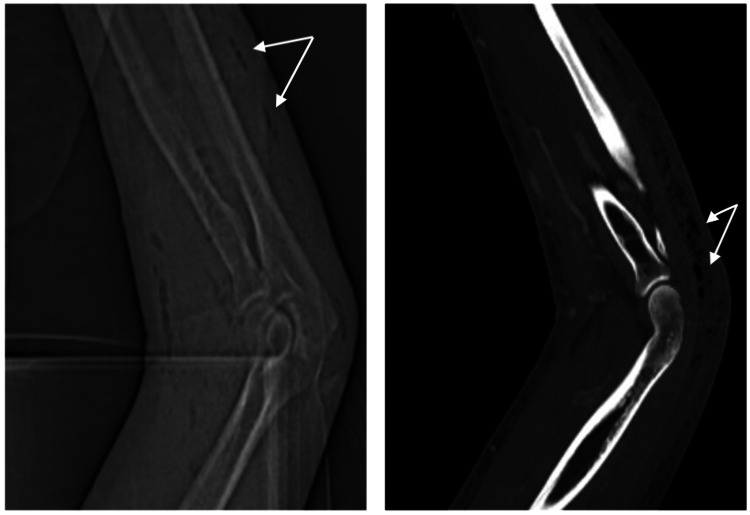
Radiographic imaging of the left upper extremity showing subcutaneous emphysema extending from the shoulder to the forearm (arrows) concerning necrotizing fasciitis.
